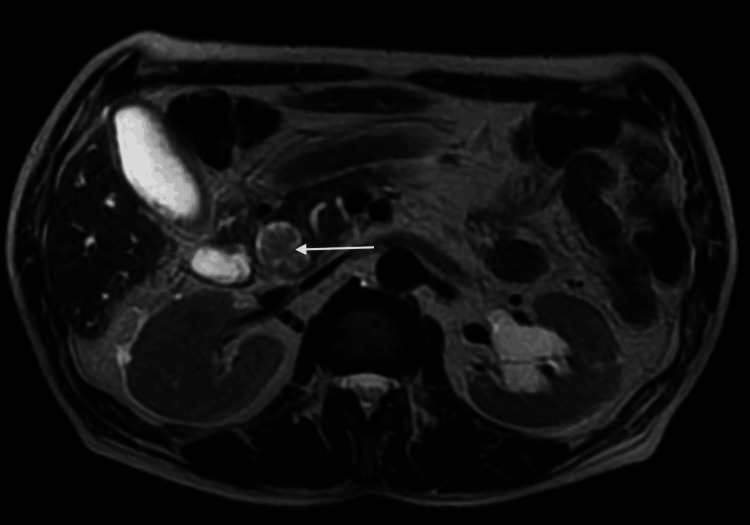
Axial T2-weighted image. Axial T2-weighted image depicts an intermediate signal lesion within the distal common bile duct (arrow). The tumor is confined to the common bile duct with an intraluminal growth. There is no sign of invasion of adjacent structures.
